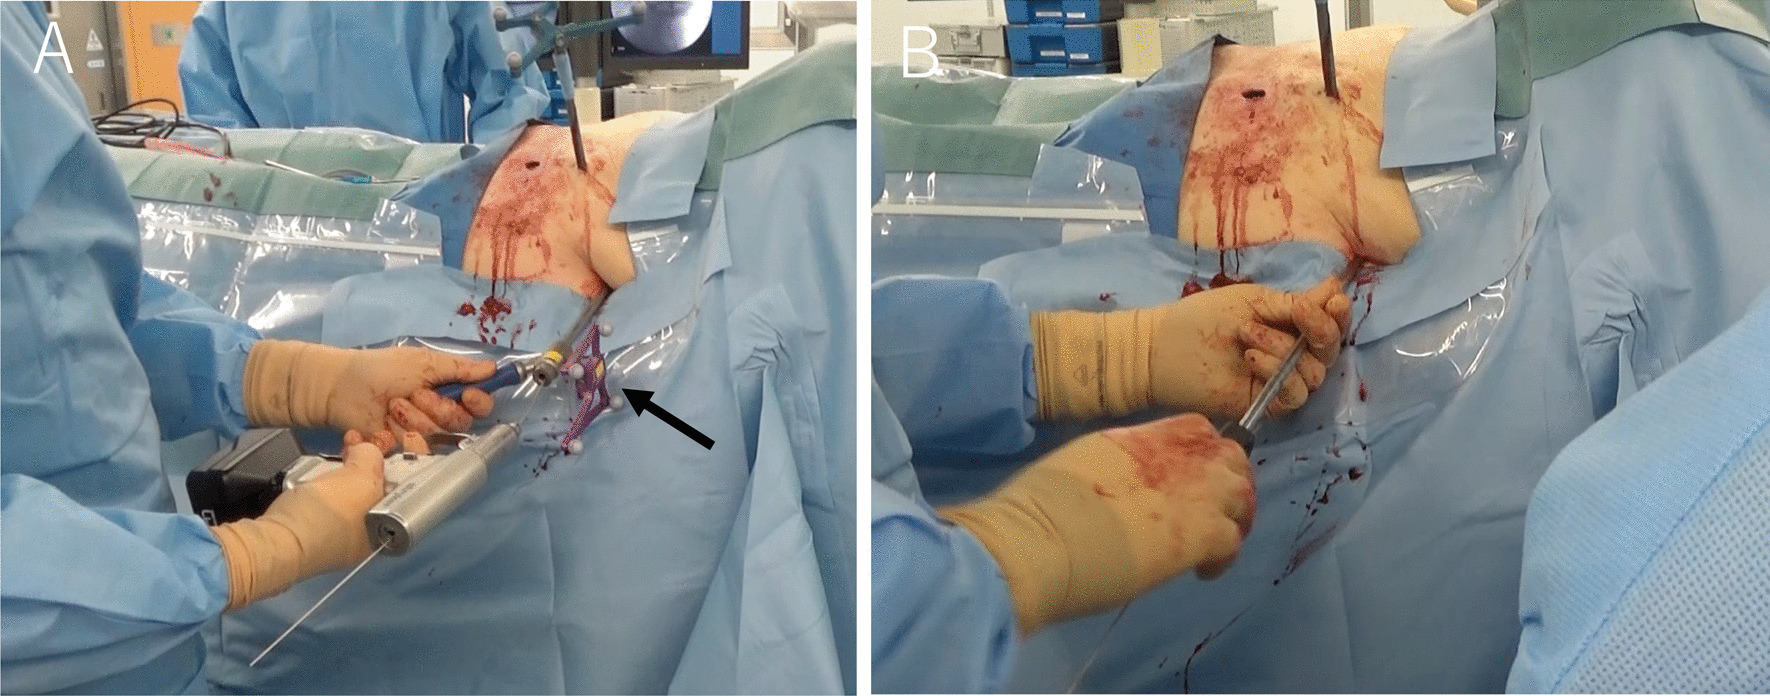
Transiliac–transsacral (TITS) screw navigation for pelvic ring fracture. The guide wire was inserted into the starting point on the fracture side using intraoperative CT navigation and Universal Cannulated Screw Set (UCSS, Medtronic) (a). The arrow indicates Universal Cannulated Screw Set. Under fluoroscopy and navigation, we confirmed that the guide wire was inserted into the iliac sacrum. We inserted the Transiliac–transsacral (TITS) over the guidewire (b).
